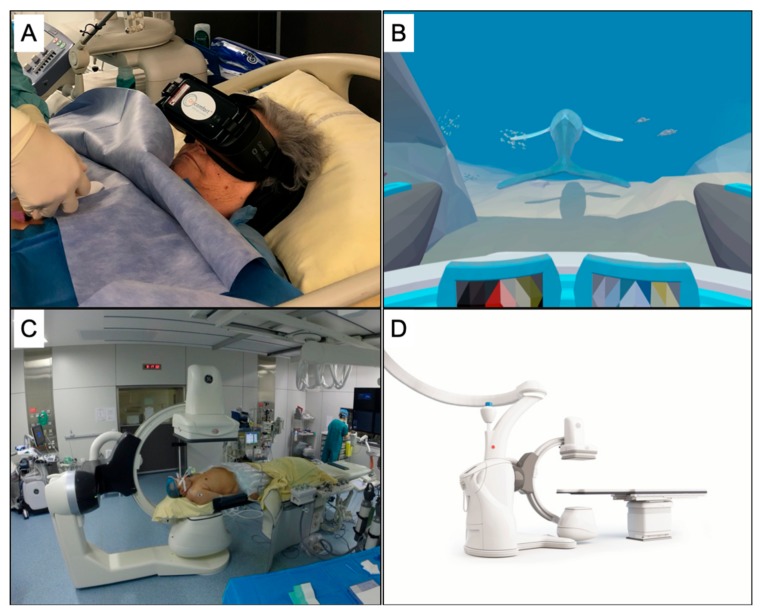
Integrative medicine in interventional oncology. (A) Digital sedation is provided by a virtual reality mask and headphones. (B) A screenshot of the digital sedation system. The patient follows a whale and has to adjust his breath according to the motion of the tail of the whale (courtesy of Oncomfort SA, Bruxelles, Belgium). (C) Interventional oncology operating rooms are often first designed to incorporate functions supporting highly advanced medical care, such as cone beam computed tomography, before considering patient well-being. (D) Future facilities using mobile robotics-assisted medical systems will allow to easily perform in-suite integrative medicine techniques and to improve the patient pathway to and from the operative rooms, limiting additional stress and anxiety (Discovery cone beam computed tomography system, courtesy of General Electric Healthcare, Buc, France).
